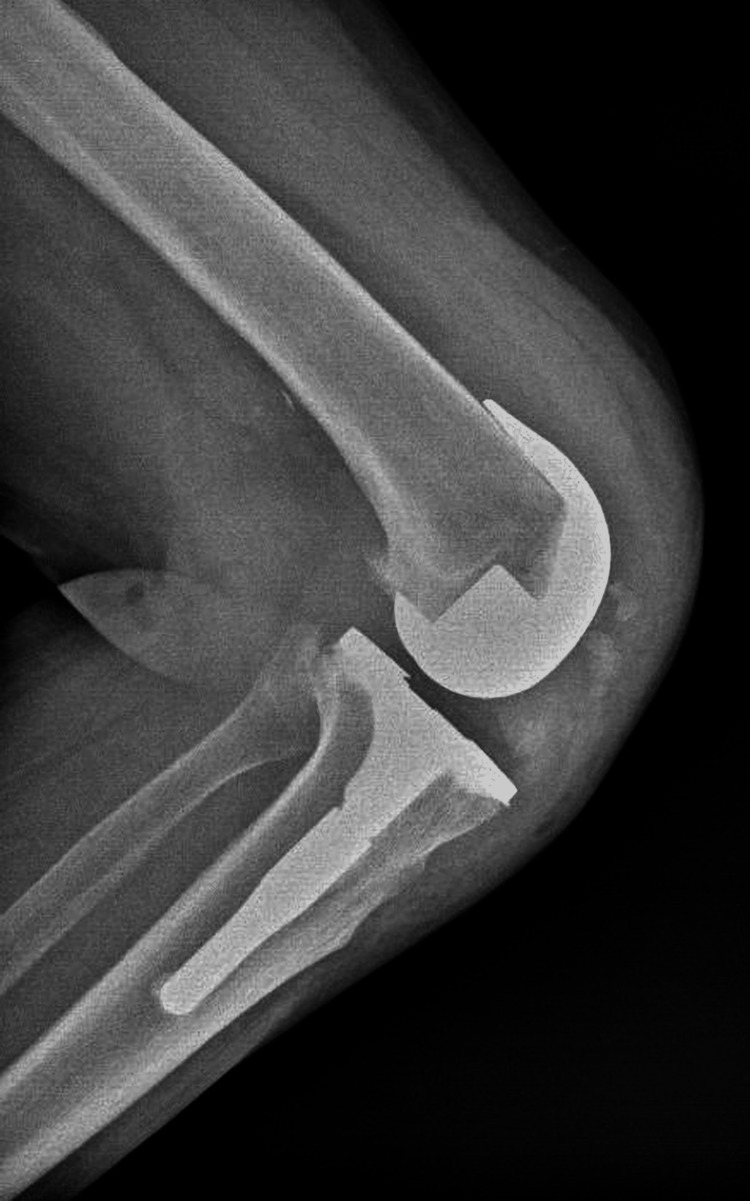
Lateral X-ray of the Left Knee at Six Months
